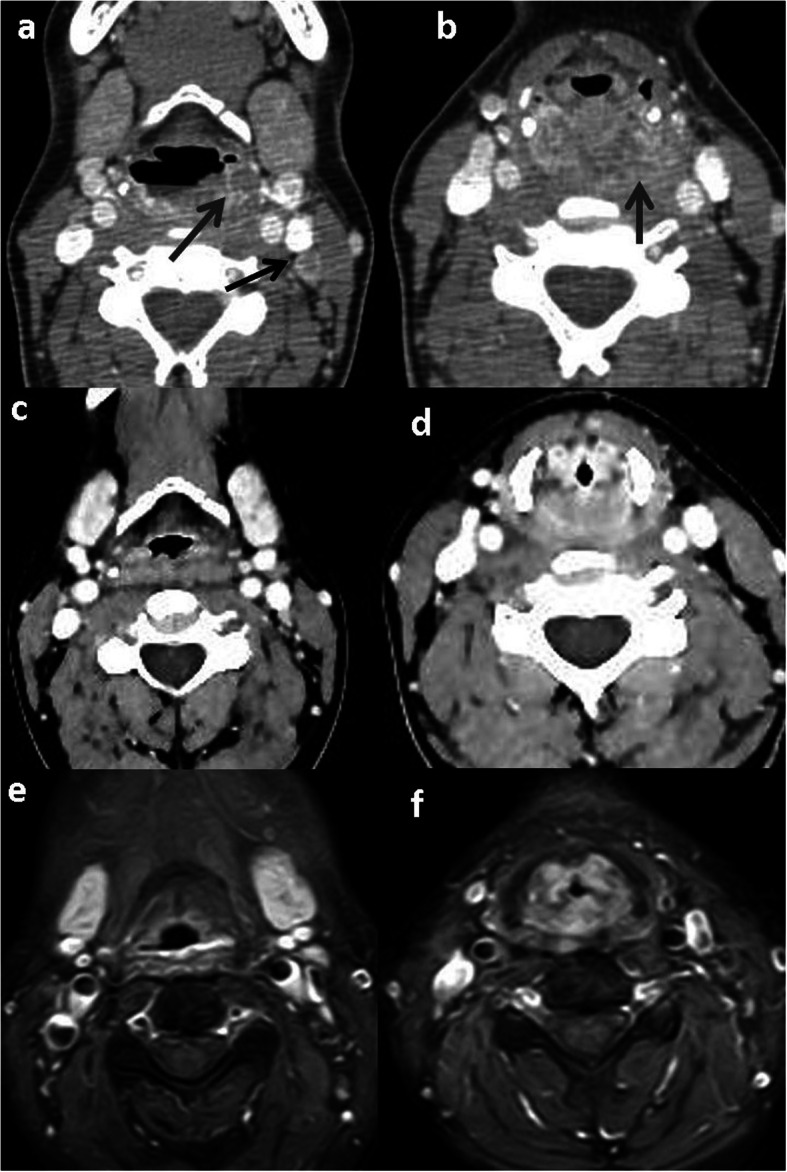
Primary NI-RADS 2, Neck NI-RADS 1 hypopharyngeal carcinoma: a, b Pre-treatment axial CECT shows hypopharyngeal SCC (grey arrows) Left malignant LN with cystic changes (black arrow). c, d first post treatment follow up CT shows diffuse hypopharyngeal thickening and enhancement. Resolution of the LN e, f Second follow up CEMRI axial images reveal stability of the Enhancement pattern
